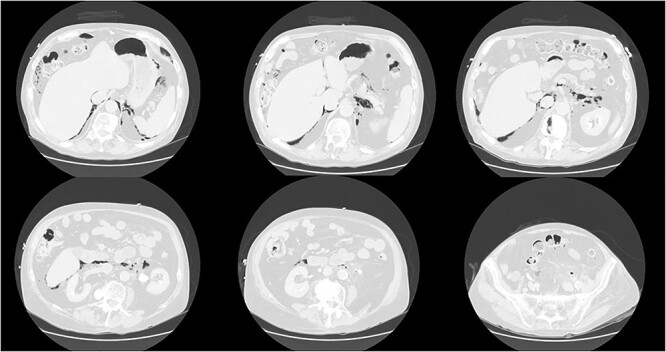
CT scan of the abdomen and pelvis demonstrating perforation of the sigmoid colon and free gas in the retroperitoneum and mediastinum.
